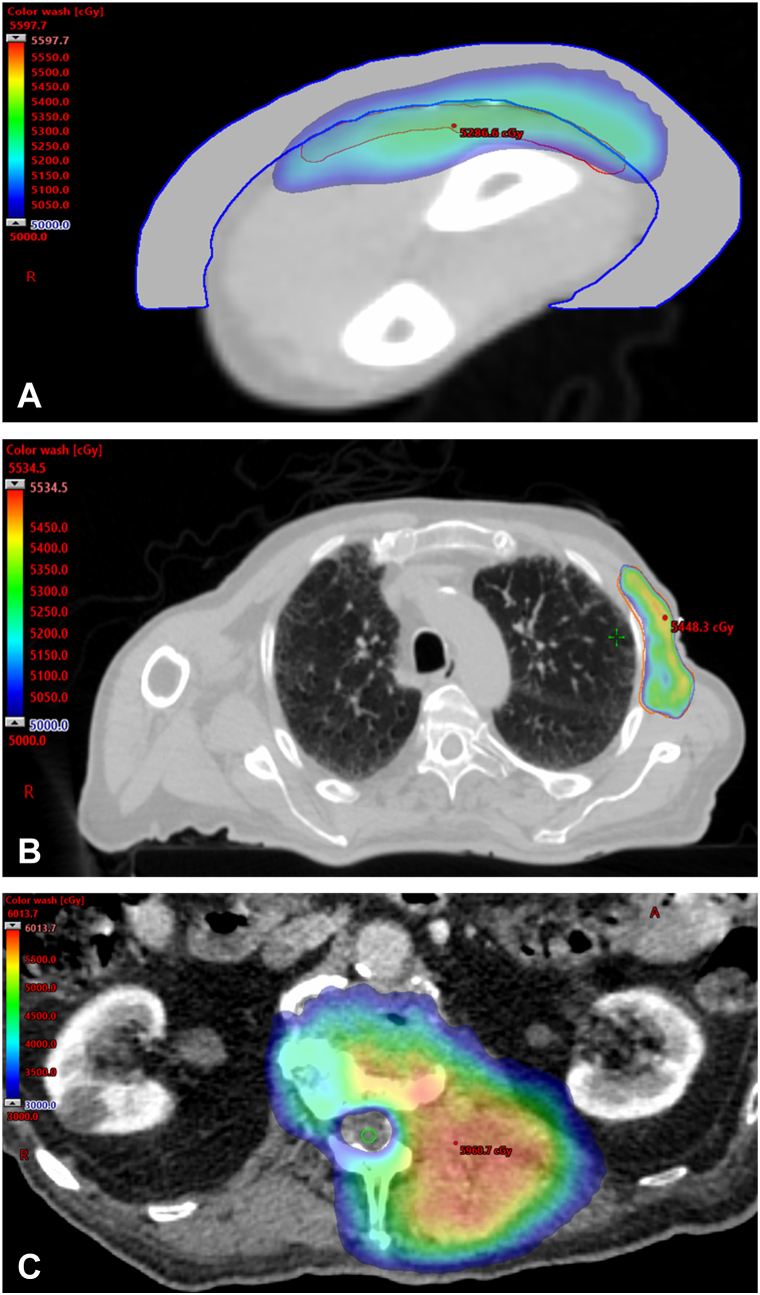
A, CT axial view of left forearm demonstrating en face electron radiation plan to a dose of 50 Gy. 1 cm bolus overlies the forearm in order to distribute dose near skin surface. B, CT axial view of chest showing highly conformal IMRT photon radiation plan for left axilla. C, CT axial view of lumbar spine demonstrating SBRT plan for L1 metastasis. Colorwash shows 30 Gy (75%) isodose line avoiding spinal cord (*green*). *CT*, Computed tomography; *IMRT*, intensity-modulated radiation therapy; *SBRT*, stereotactic body radiation therapy.
